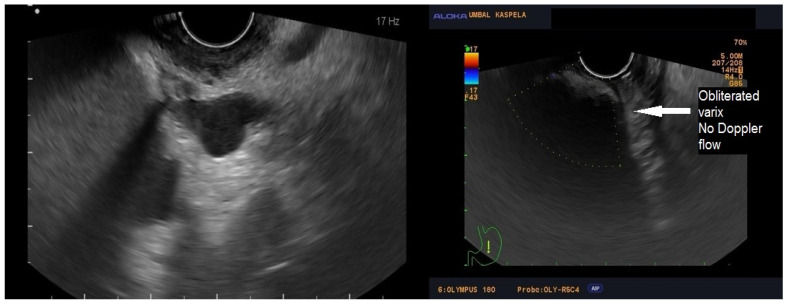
Ultrasonography showing absent Doppler flow in the treated vessel.
